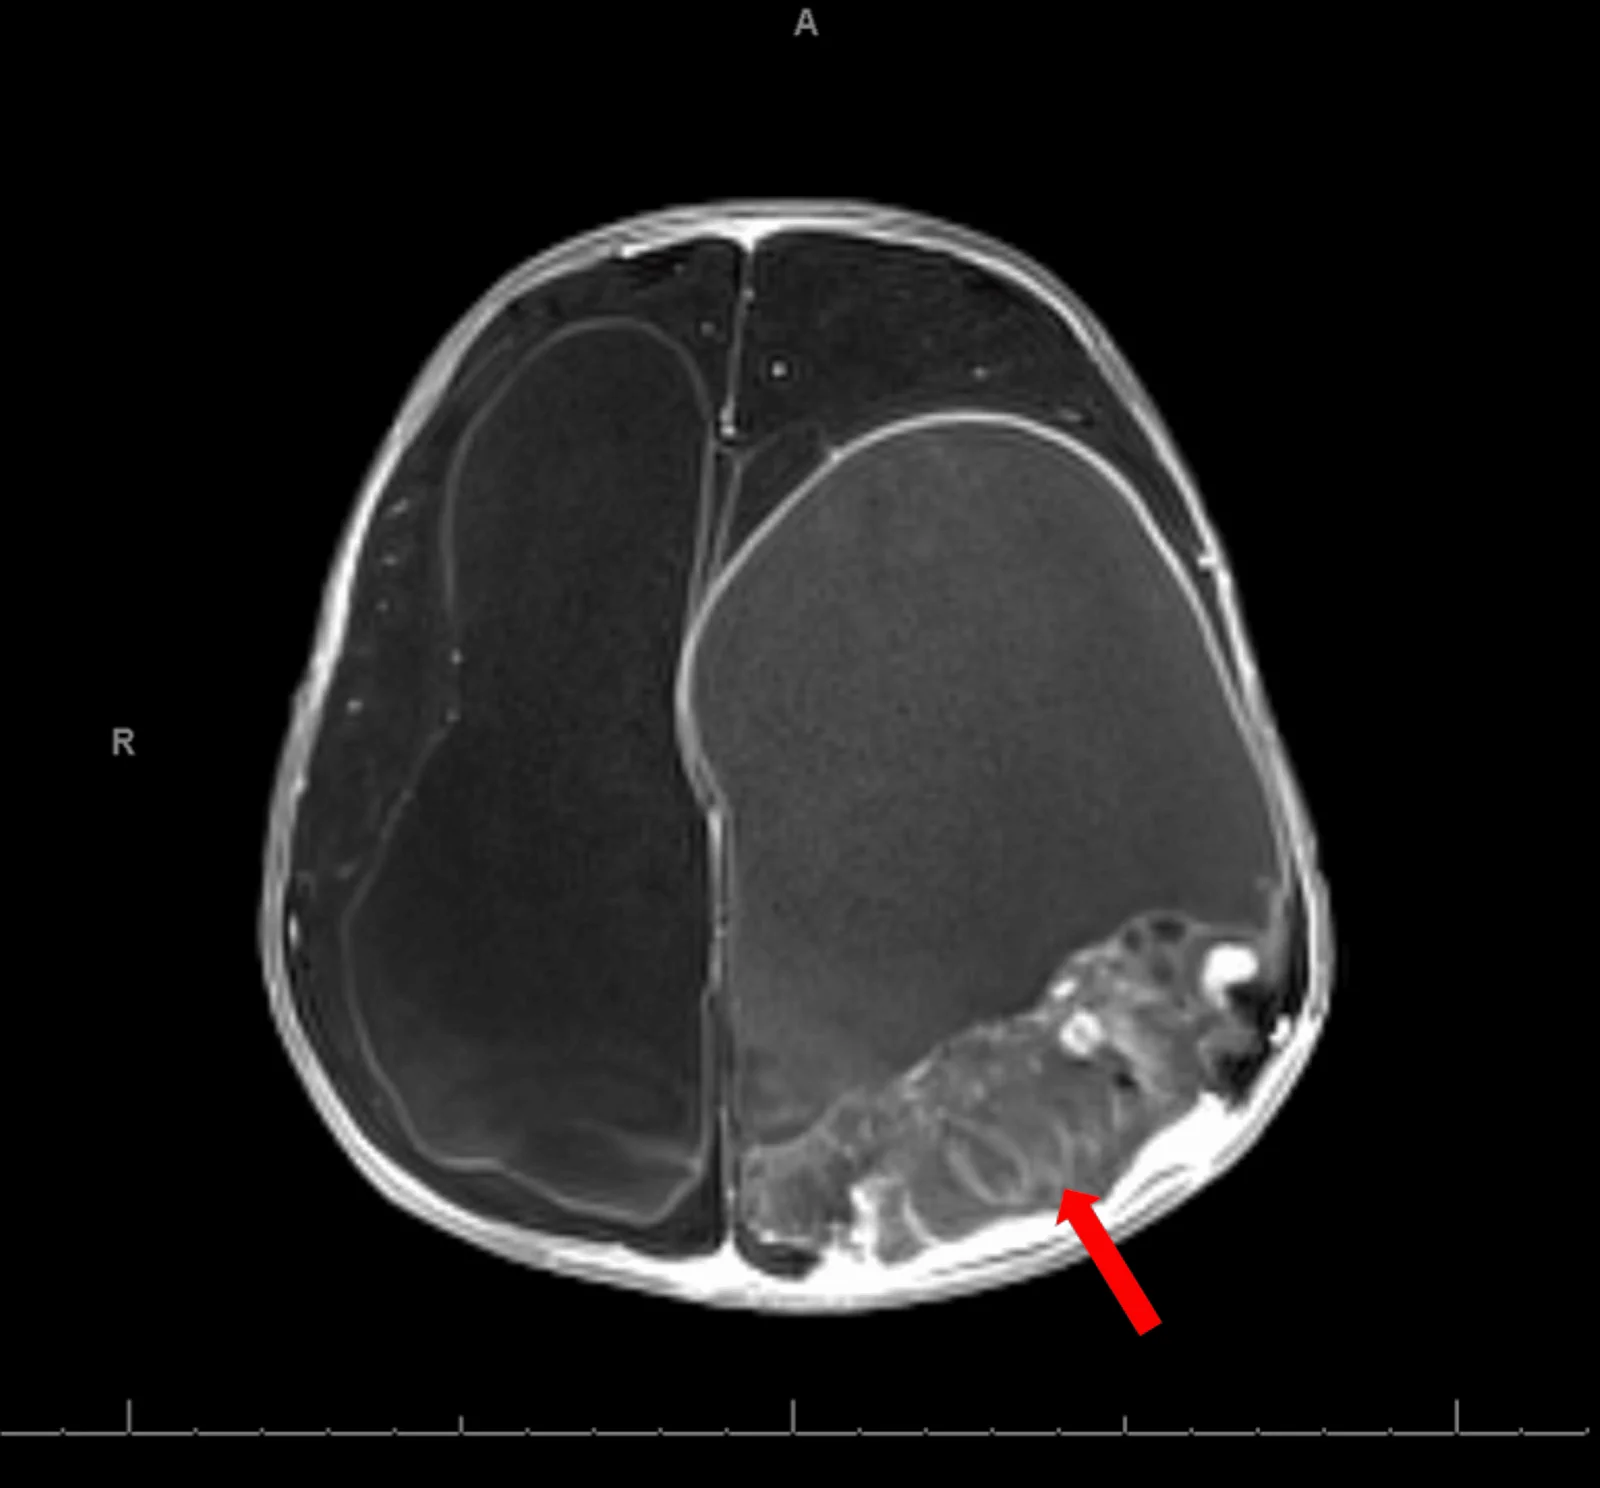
Pre-operative MRI (T1 post-contrast). Complex posterior parietal-occipital mass measuring 9.3 x 3.2 x 9.5 cm (indicated by red arrow). There is heterogeneous contrast enhancement, significant intratumoral vascularity, cystic areas, increased blood perfusion, and diffusion restriction (suggestive of a high-grade lesion). The mass causes marked right-to-left midline shift, resulting in significant mass effect on the brainstem and ventricles.
